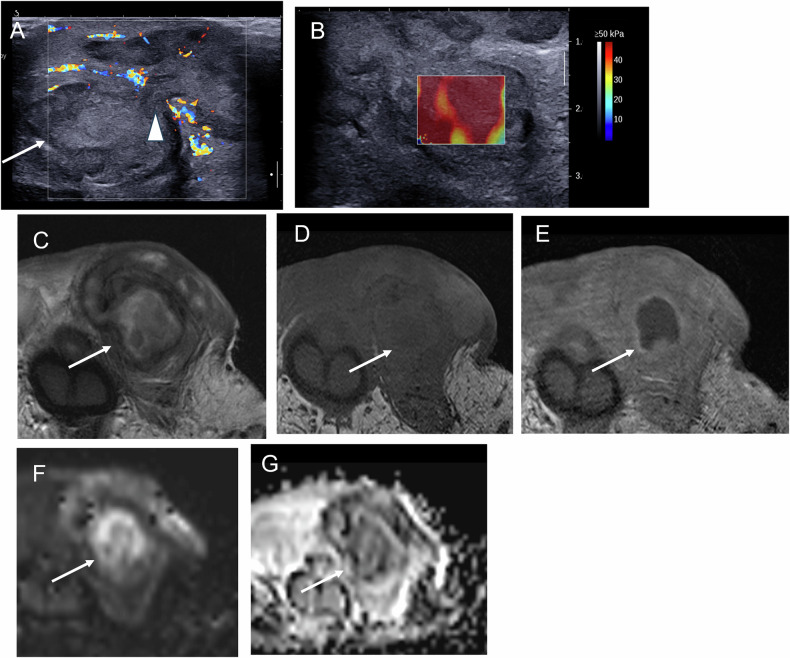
Adverse outcome of bacterial testicular infection with testicular necrosis following severe epididymo-orchitis. A Absence of vascularity on Doppler US (arrow), with fistulization (arrowhead). B Increased stiffness on SWE. C Predominant hyperintensity on T2-weighted MRI. D Absence of hyperintensity on T1-weighted image. E Peripheral rim enhancement (arrow). F, G Restricted diffusion on high b-value images and ADC map
